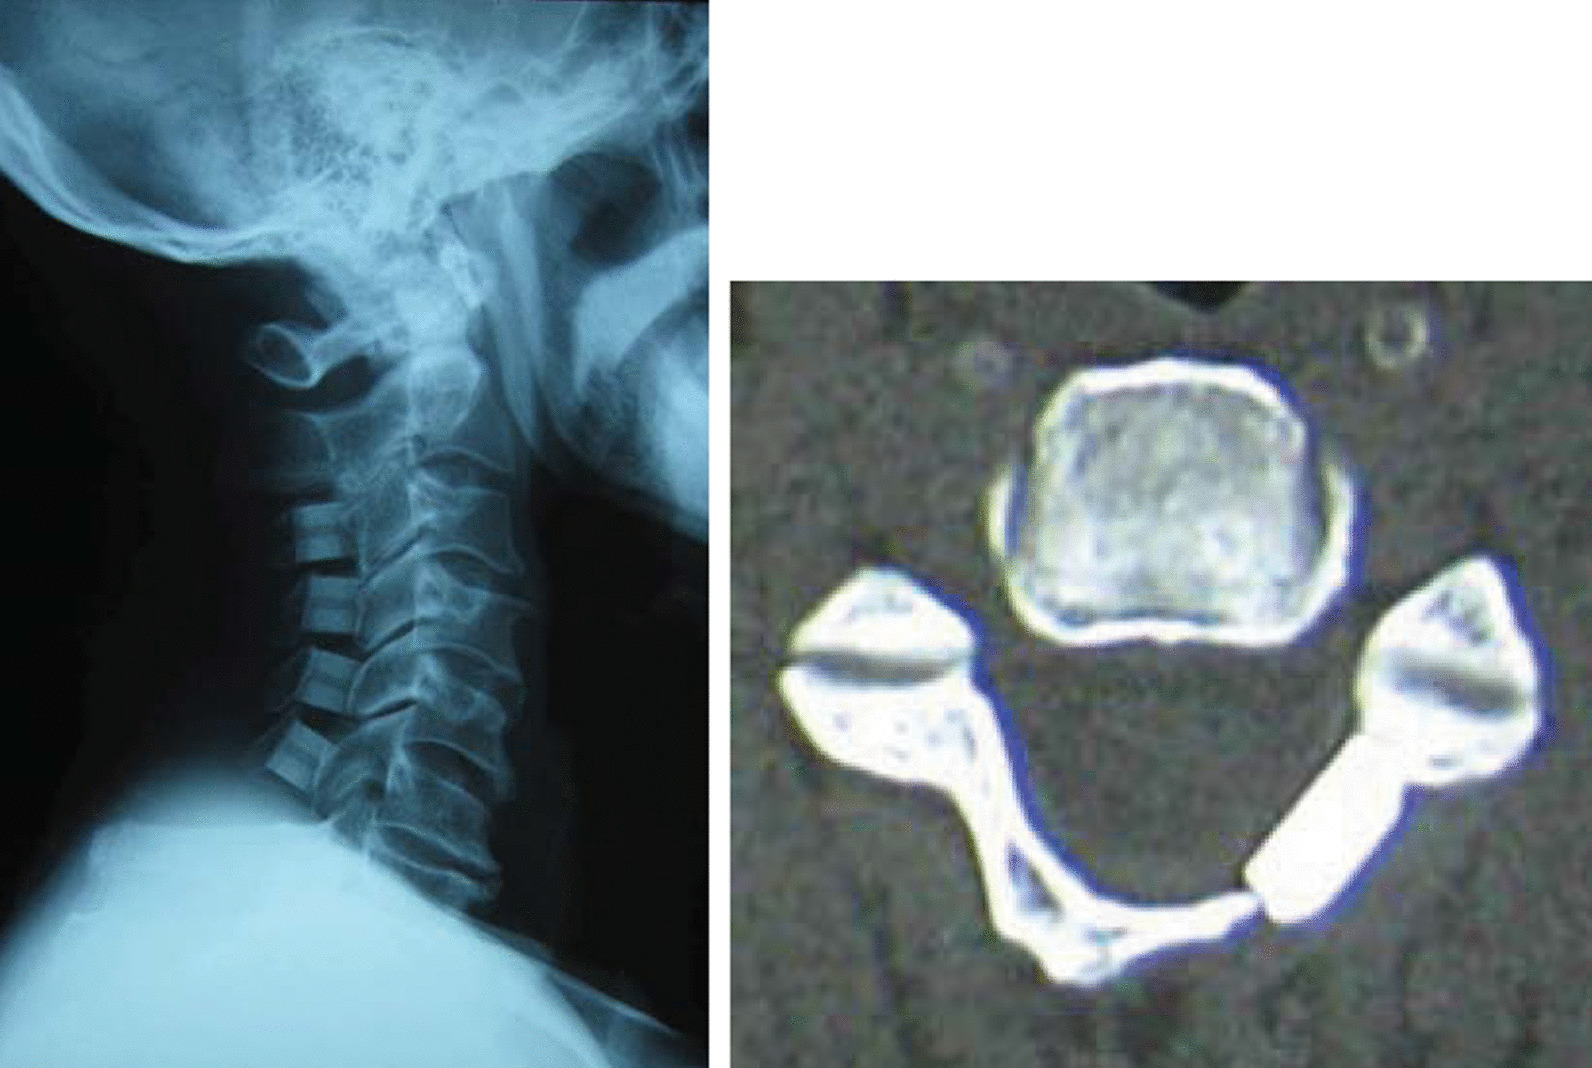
Post-operative X-rays and computed tomography images. Hydroxyapatite spacers were used for open-door laminoplasty in the present study
